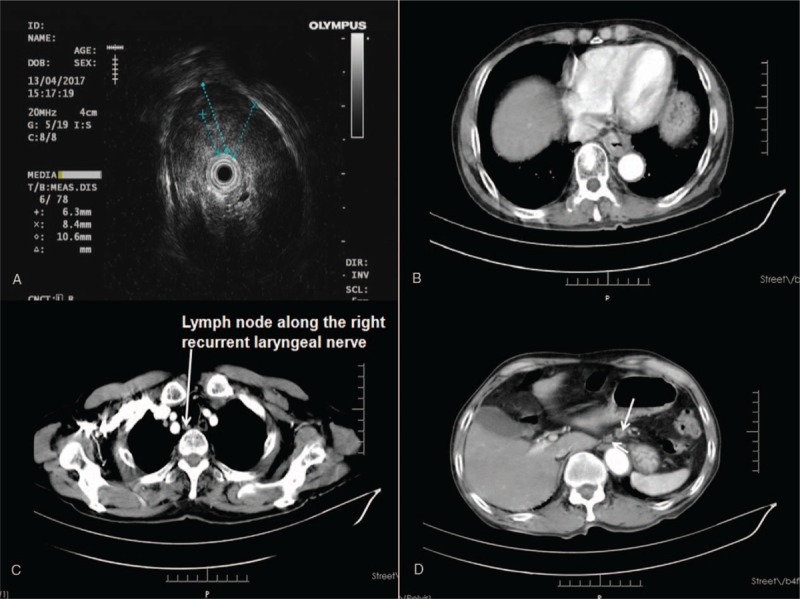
A. By endoscopic ultrasound, the esophageal tumor was staged as T3N1. B. CT scan showed wall thickening in the lower esophagus. C. A 5 mm lymph node was detected with the suspicion of metastasis along the right recurrent laryngeal nerve. D. Lymph nodes, >10 mm in diameter, were detected around the left gastric artery and gastric cardia. CT = computed tomography.
